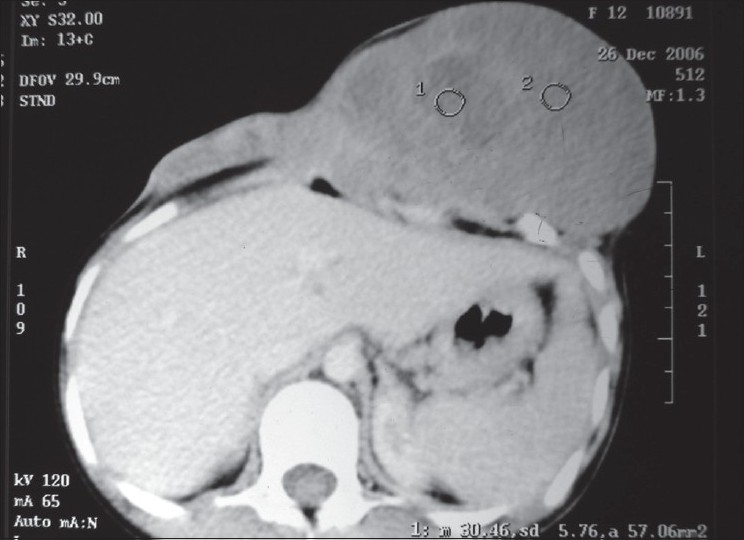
Computed tomography scan of the abdomen showing the large tumor arising from the anterior abdominal wall
